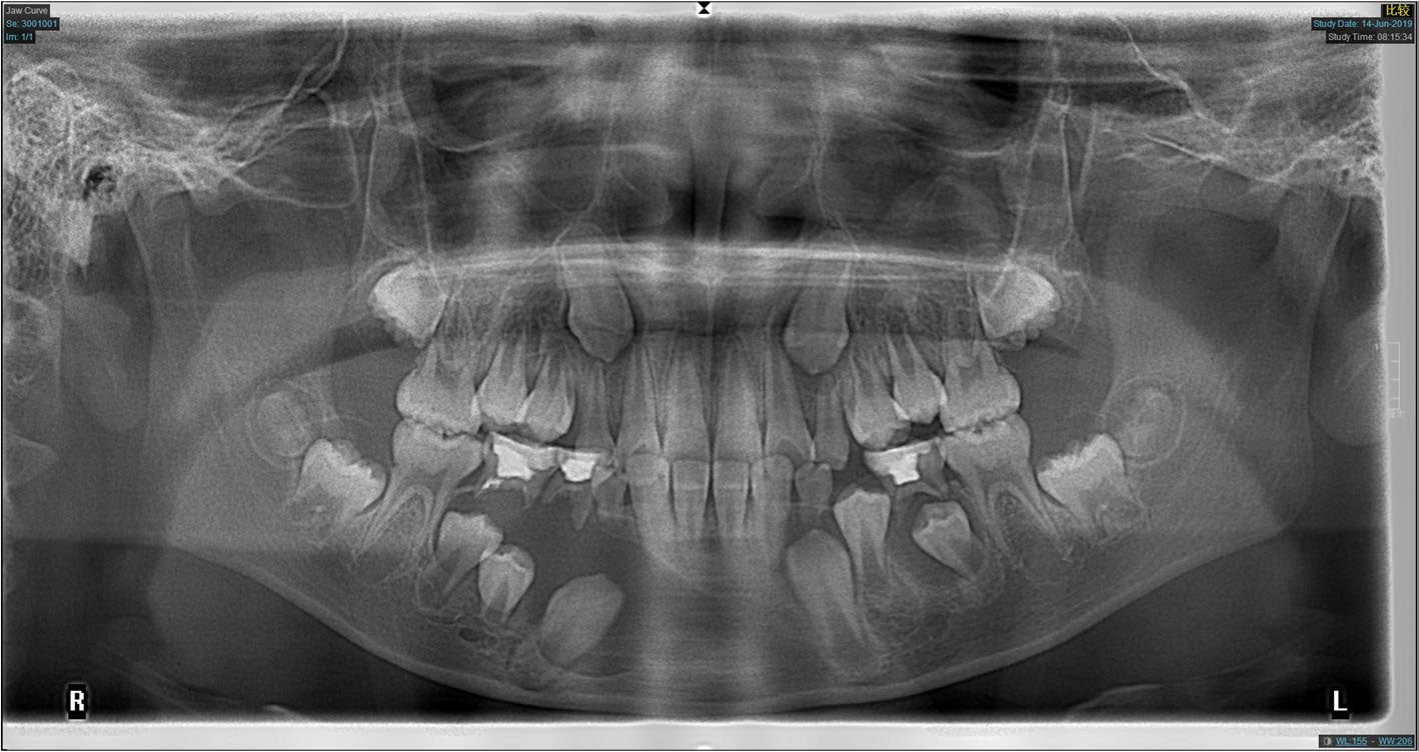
Panoramic radiograph of a 9-year-old boy at the first examination
